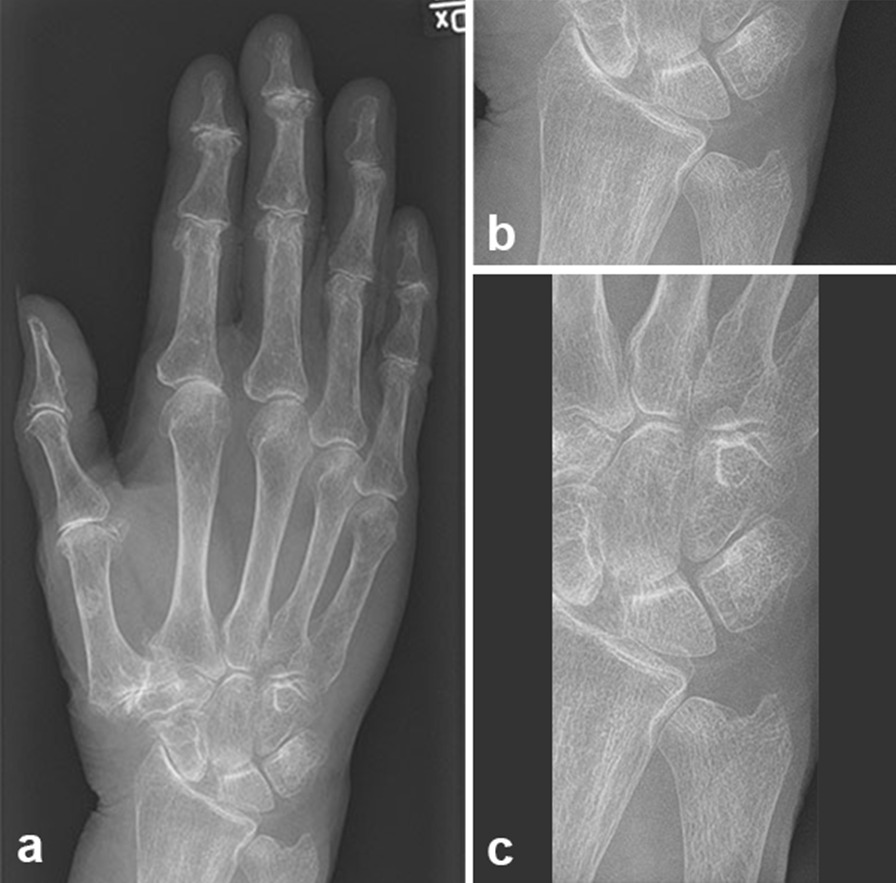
Radiograph of wrist with intact trapezium (ie, no previous surgery), before blinding (a), and after blinding (b, c)
